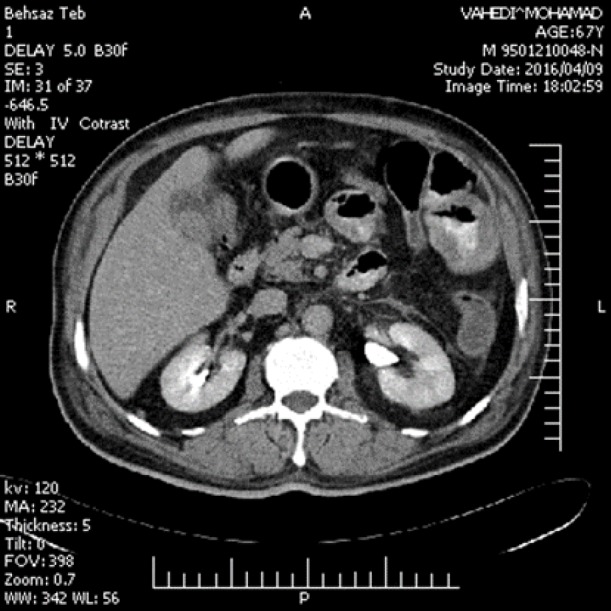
Abdominopelvic computed tomography (CT) scan with intravenous contrast; Abdominopelvic computed tomography (CT) scan with intravenous contrast revealed multiple abnormalities. CT scan exhibited an ovaloid mass in the gallbladder with washout in delayed phase that was suggestive of a tumoral lesion. There were two small nodules in the right adrenal and a heterogeneous hypodense mass (diameter: 3.2 cm) in the left adrenal. In the proximal (and to a lesser extent in distal) loops of the small intestine, a heterogeneous increase in thickness was also observed. Moreover, there were several mesenteric lymphadenopathies along the superior mesenteric artery (figure 3)
